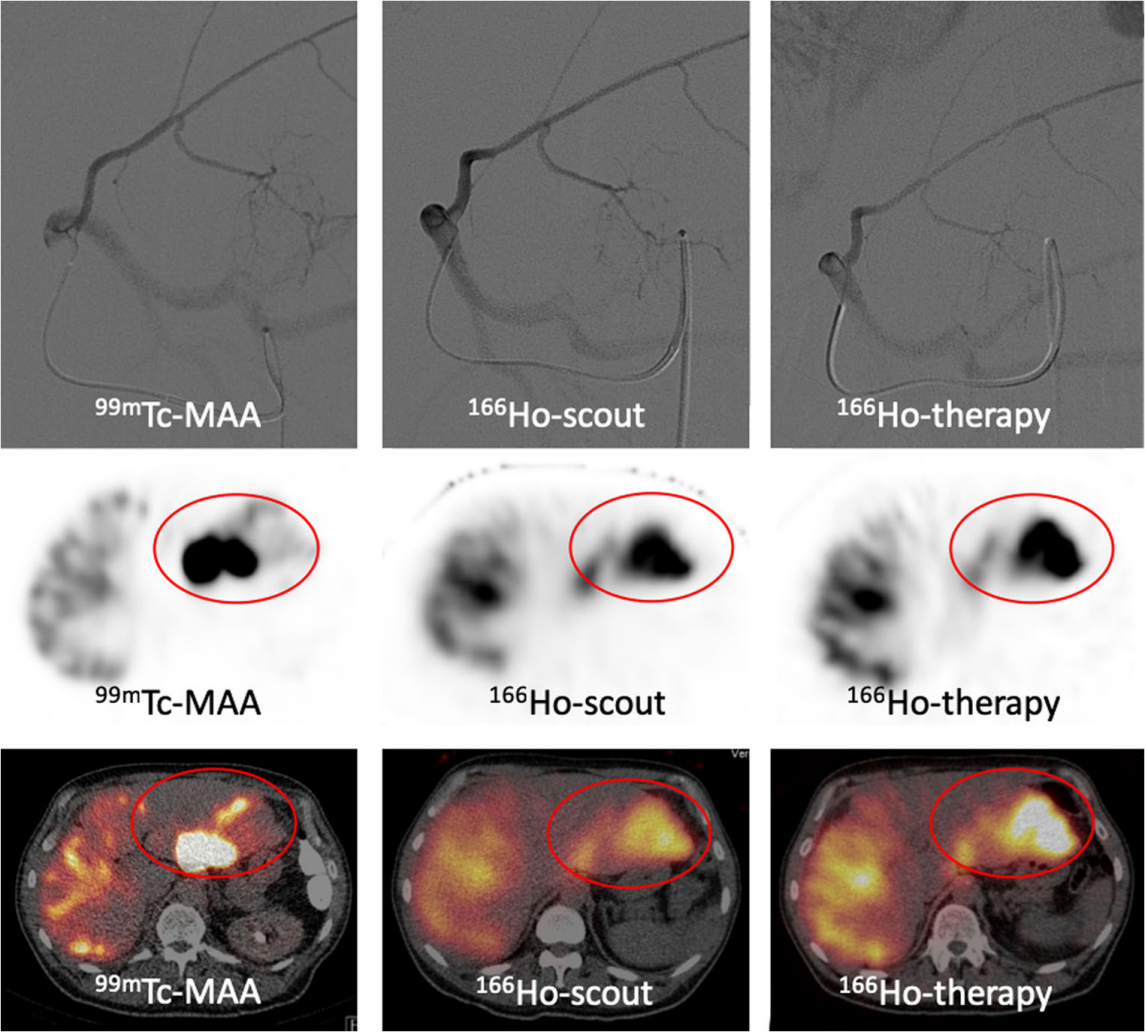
Example of discrepancy between ^99m^Tc-MAA and ^166^Ho-therapeutic dose. Despite identical catheter positions (upper row), there is a remarkable difference in activity distribution between the three procedures. SPECT-CT (middle and lower row) shows that the activity distribution in the liver of ^166^Ho-scout is more similar to the therapy distribution than ^99m^Tc-MAA. Overall agreement of ^99m^Tc-MAA was rated 2.5 compared to 4.5 for ^166^Ho scout for this patient
